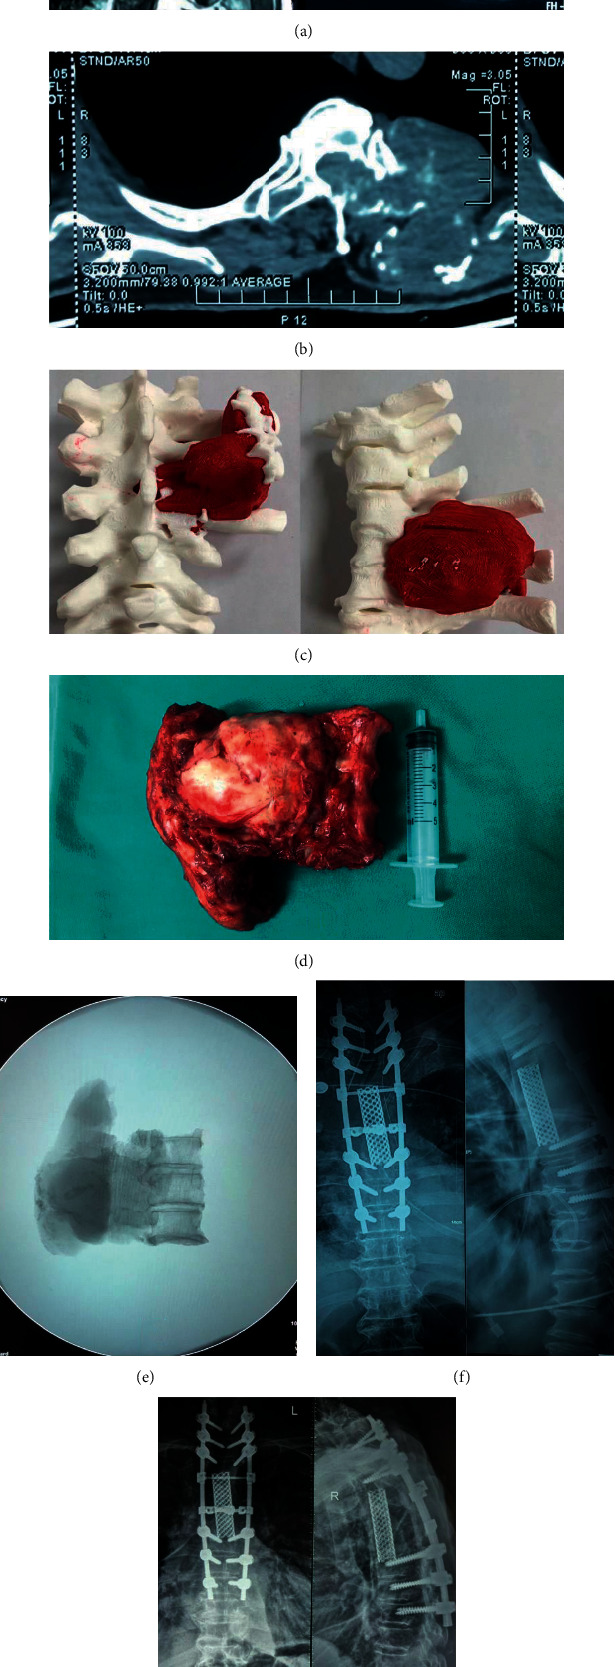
A 76-year-old male with chondrosarcoma diagnosed by needle biopsy. (a) MRI in the sagittal plane showed tumor invasion at T3, 4, and 5 levels. Transverse MRI showed that the tumor penetrated mainly to the left side of the vertebrae with left nerve root involved. The tumor showed low signal in T1 phase and medium high signal in T2 phase. (b) CT showed significant bone destruction. (c) The 3D printed model showed in detail the location and size of the tumor, the extent of its invasion in the bone tissue and its anatomic relationship with surrounding structures. (The tumor is in red). (d) The tumors completely removed during the operation were consistent with the 3D printed model in morphology, position, and other aspects. The capsule of the tumor was not destroyed. (e) On X-ray, we can see the gross structure of the lesion. The vertebrae and tumor were removed completely. (f) Postoperative X-ray showed complete removal of the lesion and good internal fixation. (g) Radiographs showed no local recurrence and solid internal fixation one year after surgery.
